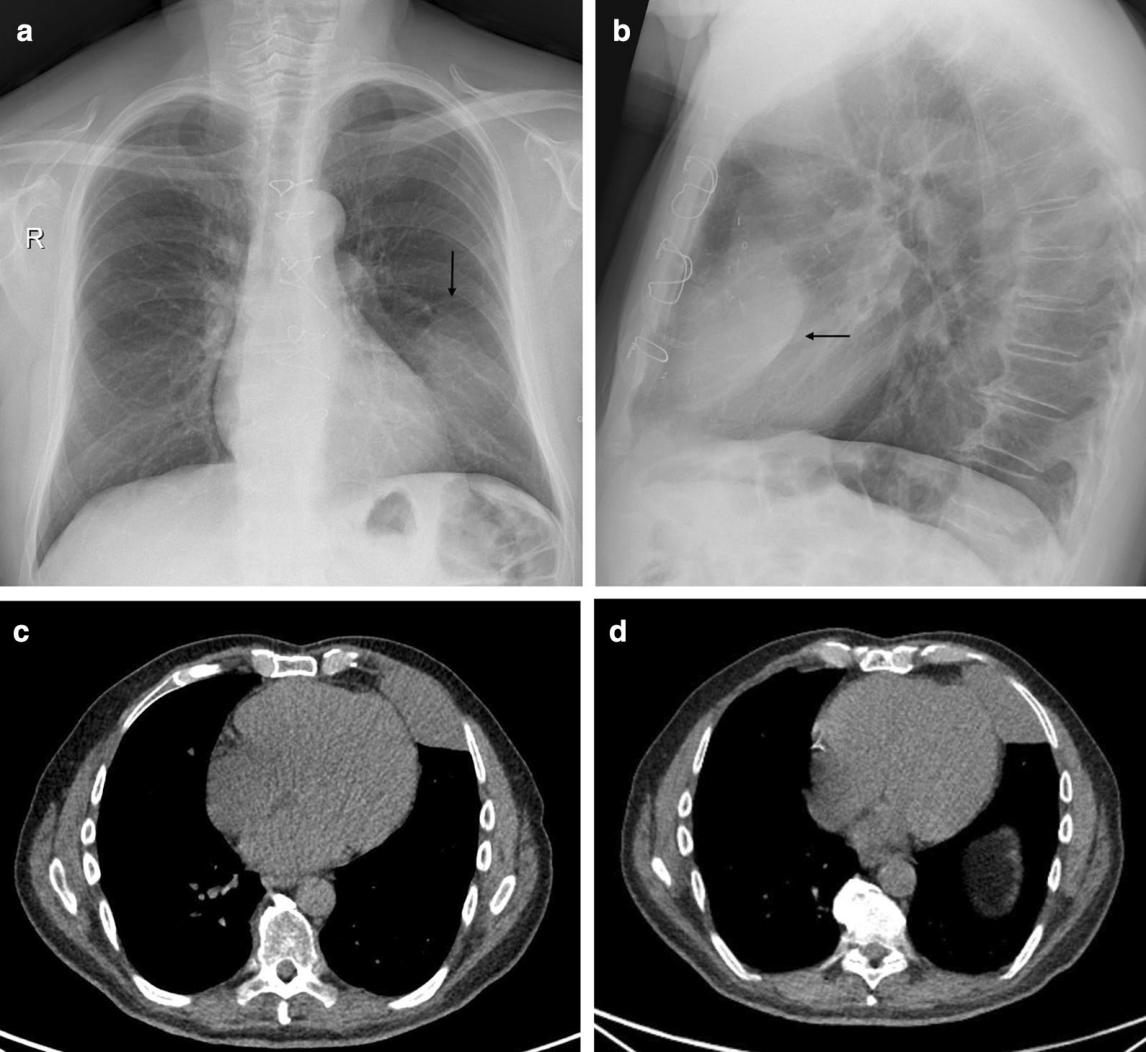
Diagnosis: desmoid tumor. Technique: standard chest radiography and chest CT. Description: A 76-year-old man (never smoker) presented with dyspnea and chronic cough. Postero-anterior and lateral chest radiographs (a, b) show a relatively ill-defined area of consolidation (arrow) in the left lung (lingular region). Axial non-contrast-enhanced CT (c, d) shows a relatively well-defined oval-shaped pleural-based mass, isodense to muscle. There are no signs of associated chest wall involvement. Diagnosis of a desmoid tumor was made after surgical resection
